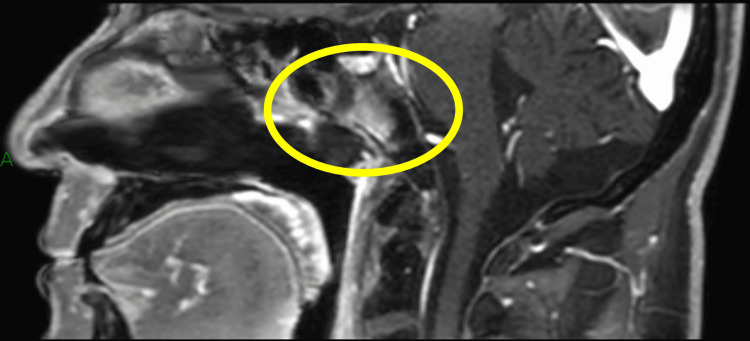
Magnetic resonance imaging one month after photoimmunotherapy One month after photoimmunotherapy, magnetic resonance imaging showed contrast enhancement at the clivus, which was suspected to be osteomyelitis.
